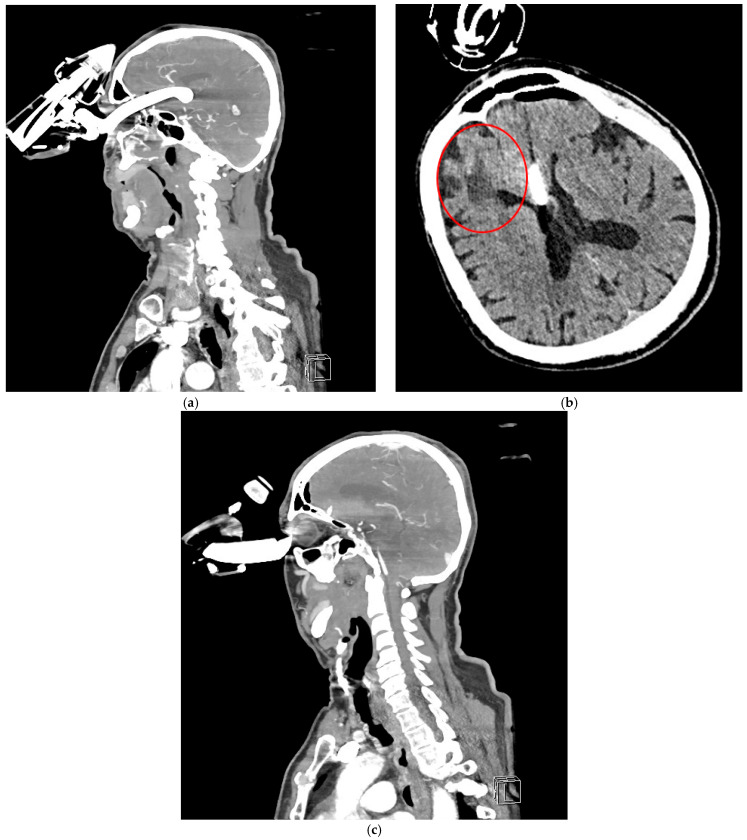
CTA revealed a foreign body penetrating the left orbit into the anterior cranial fossa, extending into the third ventricle to a depth of approximately 64 mm (a). The anterior cerebral arteries were visualized along the foreign body, both running on its left side. No significant intracranial hemorrhage was observed. Additionally, features of porencephaly were noted, likely resulting from a previous injury to the right frontal lobe (red circle—the patient had a history of two head CT scans at our hospital, in 2012 and 2014, which showed evidence of porencephaly) (b). The ventricular system was normal in size and position, without displacement or enlargement. (c) The left eyeball was displaced laterally and inferiorly, compressed by the foreign body, but showed no evidence of direct injury.
